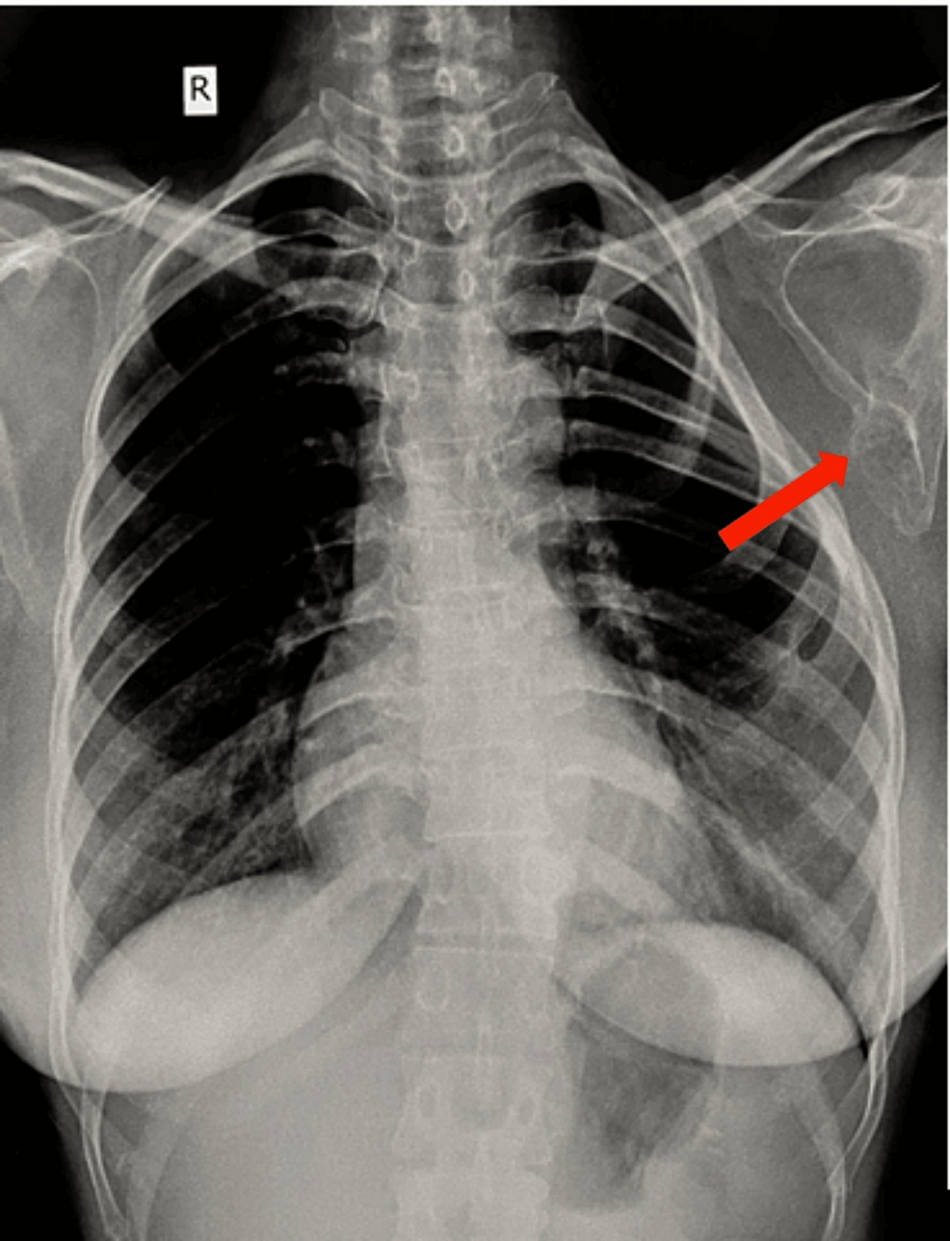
Postoperative X-ray after 6 months showing corrected chest deformity
